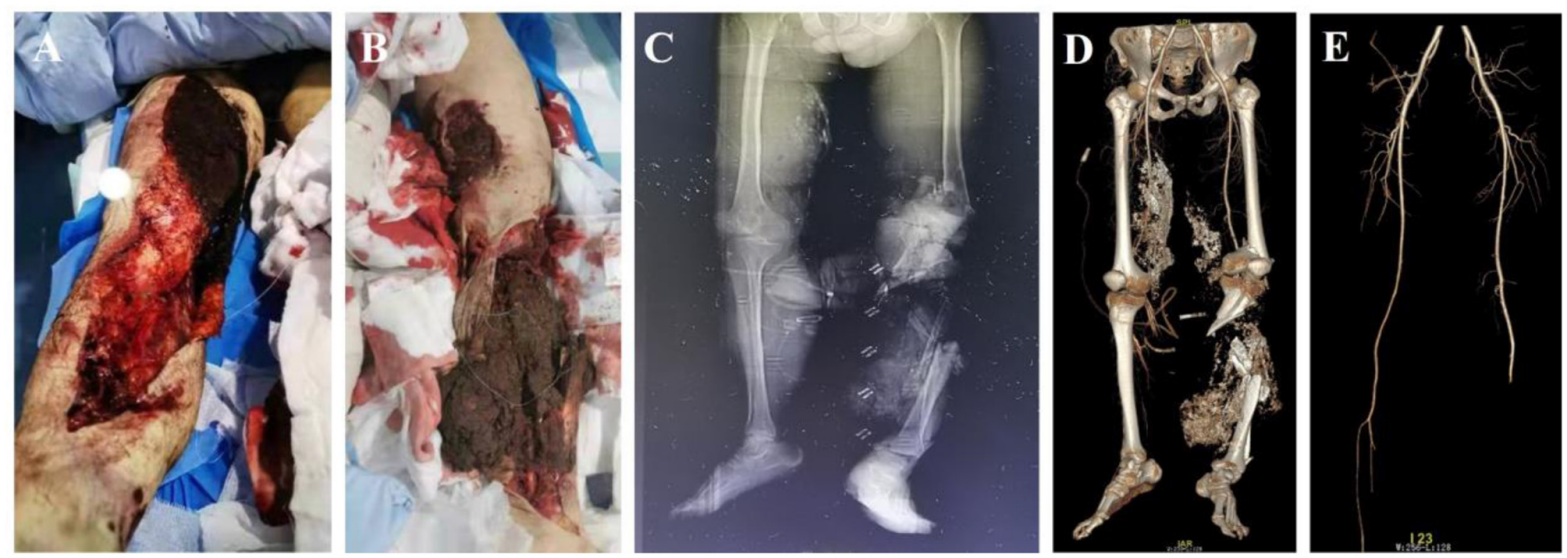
Critical Patient 8, diagnosis: blast-induced combined injury and multiple trauma (ISS 50 points: a tracheotomy and other surgeries were performed 35 h after the injury. Subsequently, damage control surgeries were conducted in 6 stages, and the patient was discharged after 37 days of treatment). (A) Open injury to the right lower limb. (B) Destructive injury to the left lower limb. (C, D). X-ray and CT reconstruction of the pelvis and bilateral lower limbs reveals a comminuted fracture of the left tibia and fibula with vascular damage. (E) CTA of the bilateral lower limbs shows injury to the left calf artery.
